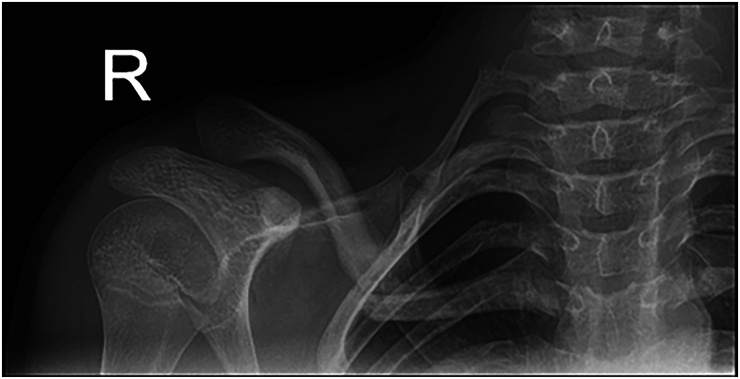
X-ray following re-injury showing a complete fracture of the right clavicle
